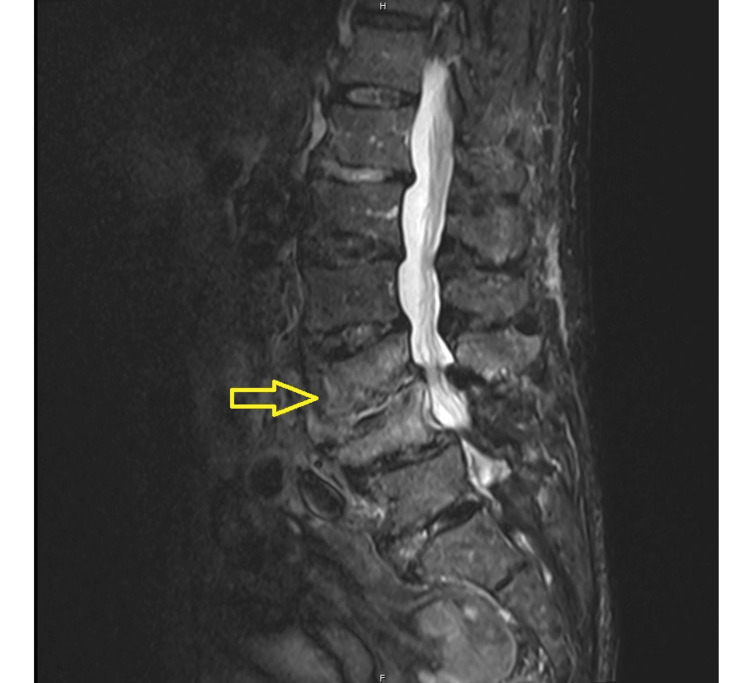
Sagittal contrast-enhanced MRI of the lumbar spine Arrow highlights diffuse marrow edema in the L3 and L4 vertebral bodies with decreased intervertebral disc space. There is enhancing ventral epidural tissue at L3-4 without discrete non enhancing collection. These findings are suggestive of discitis osteomyelitis without discrete abscess.
